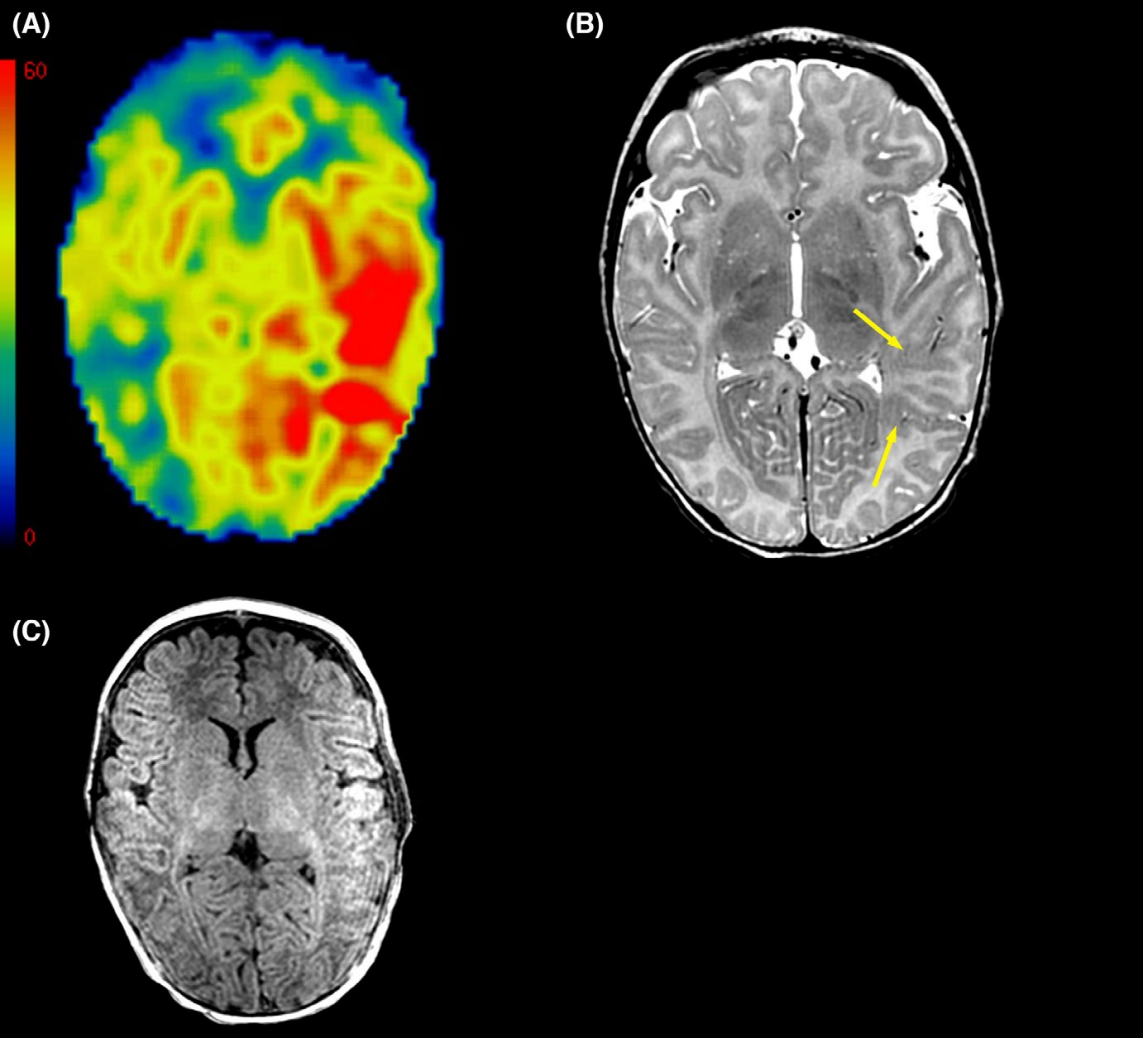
Case 3: Eight‐week‐old with left posterior temporal FCD IIa. Of note, this MRI was initially interpreted as non‐lesional. (A) 3D pseudo‐continuous ASL MRI showing hyperperfusion (bright red) in the region of left posterior temporal FCD. This FCD is much larger than Cases 1 and 2, which is why there is a broader extent of hyperperfusion. Scale is mL/100 g/min. Slice thickness 3 mm, Delay time 1025 ms, pixel size 0.22 mm^2^. (B) Axial T2 showing subtle thickening, poor gray‐white differentiation (yellow arrows), showing the utility of the ASL hyperperfusion in difficult cases. (C) On Axial T1, the lesion is not appreciated.
